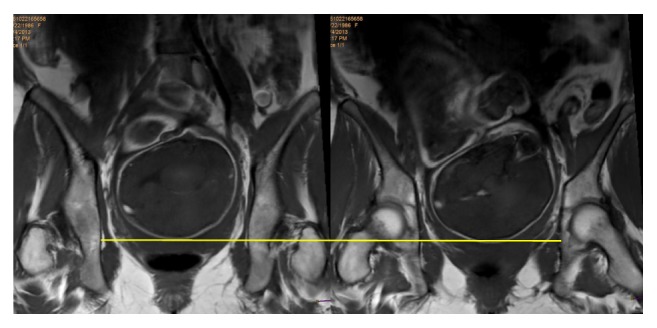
MRI coronal planes showing the fetal head at the level of the ischial spine.
